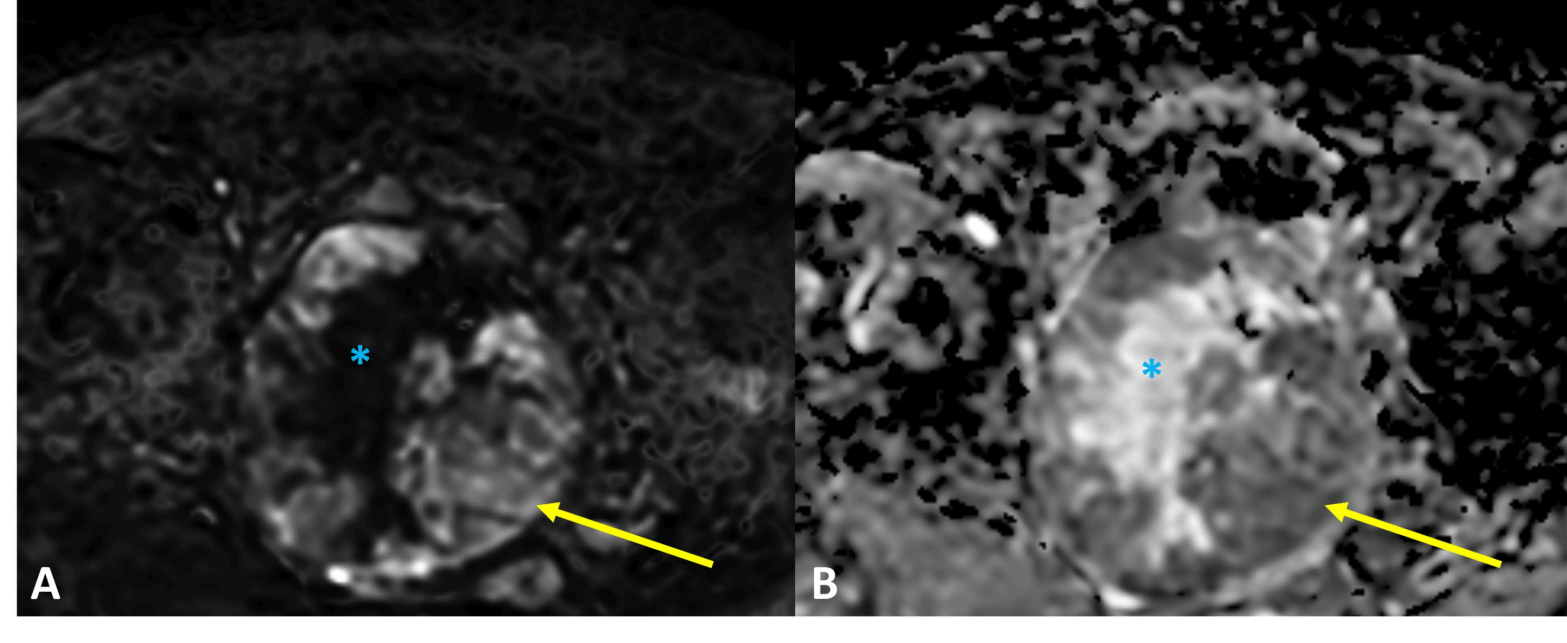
Diffusion-weighted and ADC images of the uterine inflammatory myofibroblastic tumor. Axial diffusion-weighted imaging (DWI) at b = 1000 (A) and the apparent diffusion coefficient (ADC) map (B) show low ADC values in the solid component (yellow arrow), consistent with true restricted diffusion, and higher ADC values centrally (blue asterisk) within the necrotic area shown in Figure 1, consistent with facilitated diffusion.
